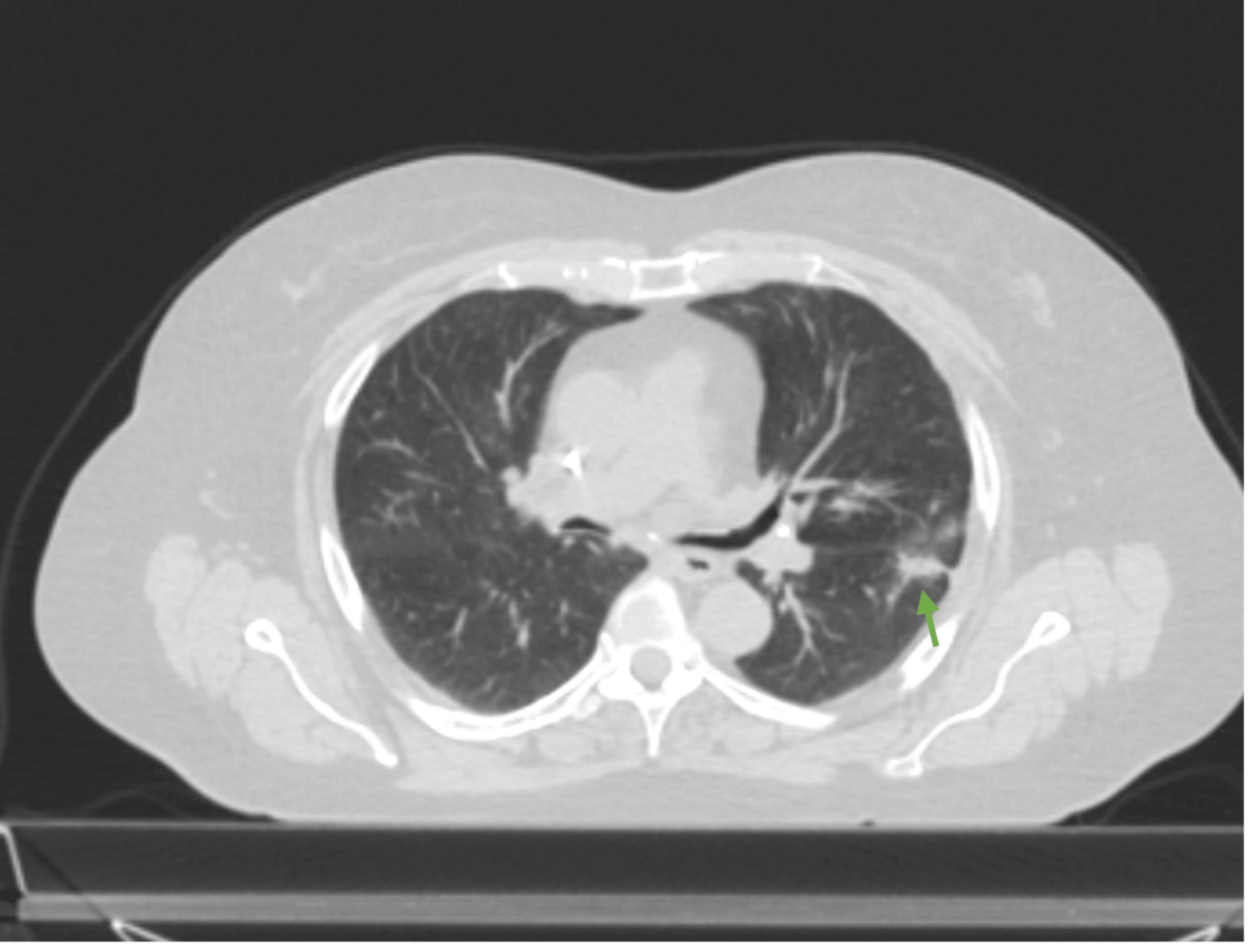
Axial view CT chest without contrast performed for radiation staging All nodules/masses are nearly resolved (green arrow). Slice thickness: 2mm. Edge-enhanced reconstruction algorithm.
